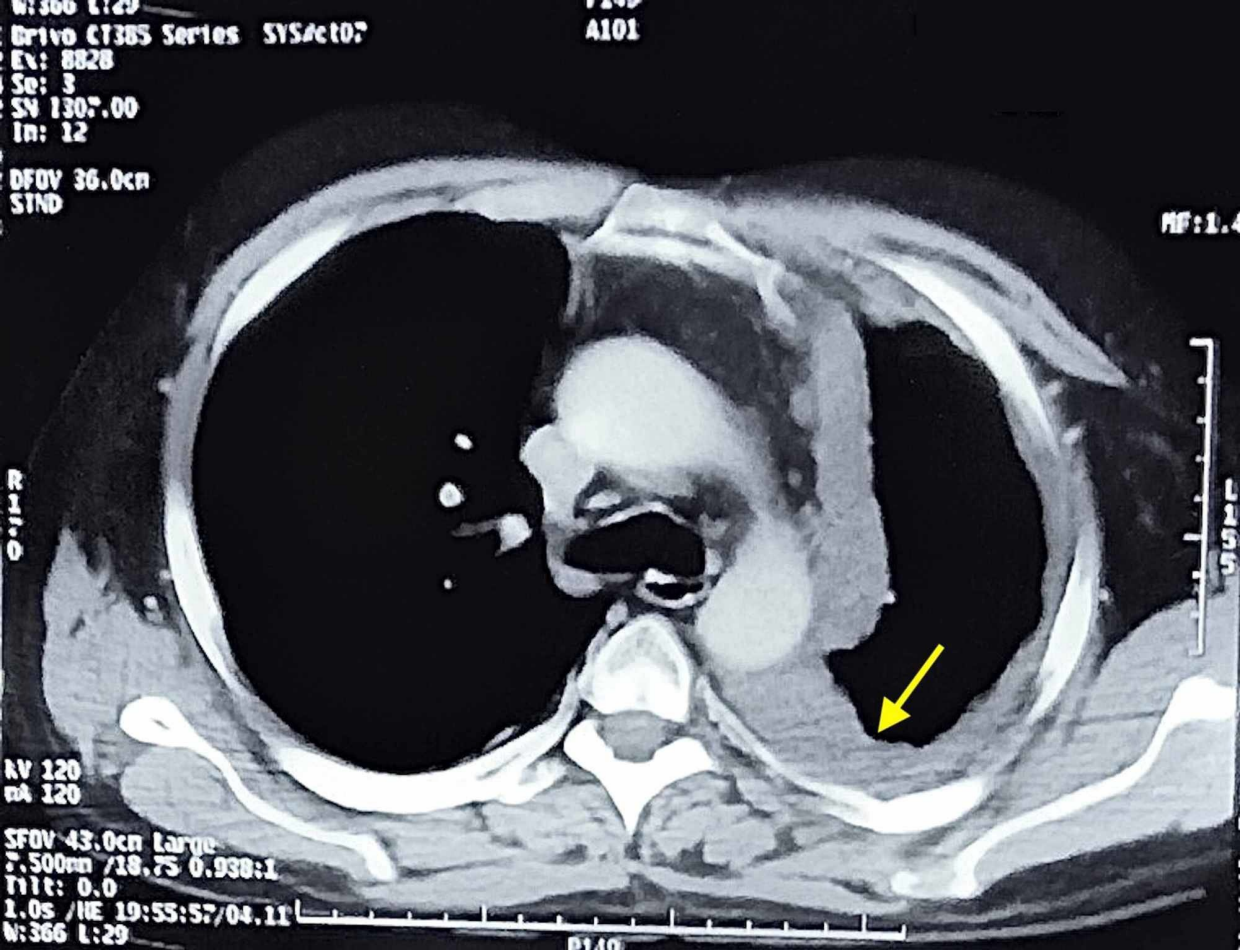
A chest CT showing residual circumferential hypodense pleural thickening involving the mediastinal, costal and diaphragmatic pleural surfaces. Erosion of the inner aspect of multiple ribs is noted. Left lung is inflated with fibroelastic bands (yellow arrow), indicative of stage IV mesothelioma. CT: computed tomography.
